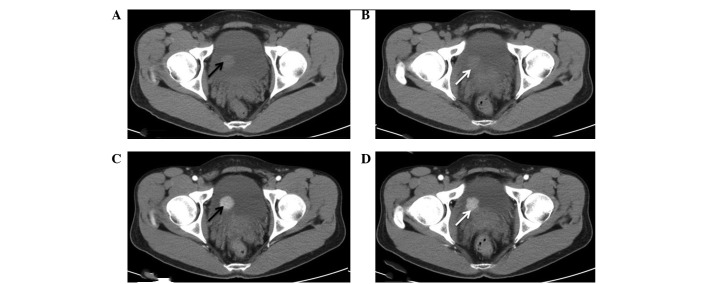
Computed tomography (CT) scanning revealed a 26×22×25 mm polypoid lesion protruding into the bladder cavity (black arrow) with a pedicle (white arrows) connected to the right postero-lateral wall of the urinary bladder, well-defined borders and no thickening of the wall near the tumor. (A and B) On the plain CT images, the mass demonstrated homogeneous iso-density and (C and D) on the contrast-enhanced CT images, homogeneously significant enhancement was observed. The degree of enhancement of the tumor was significantly higher than that of the bladder wall.
